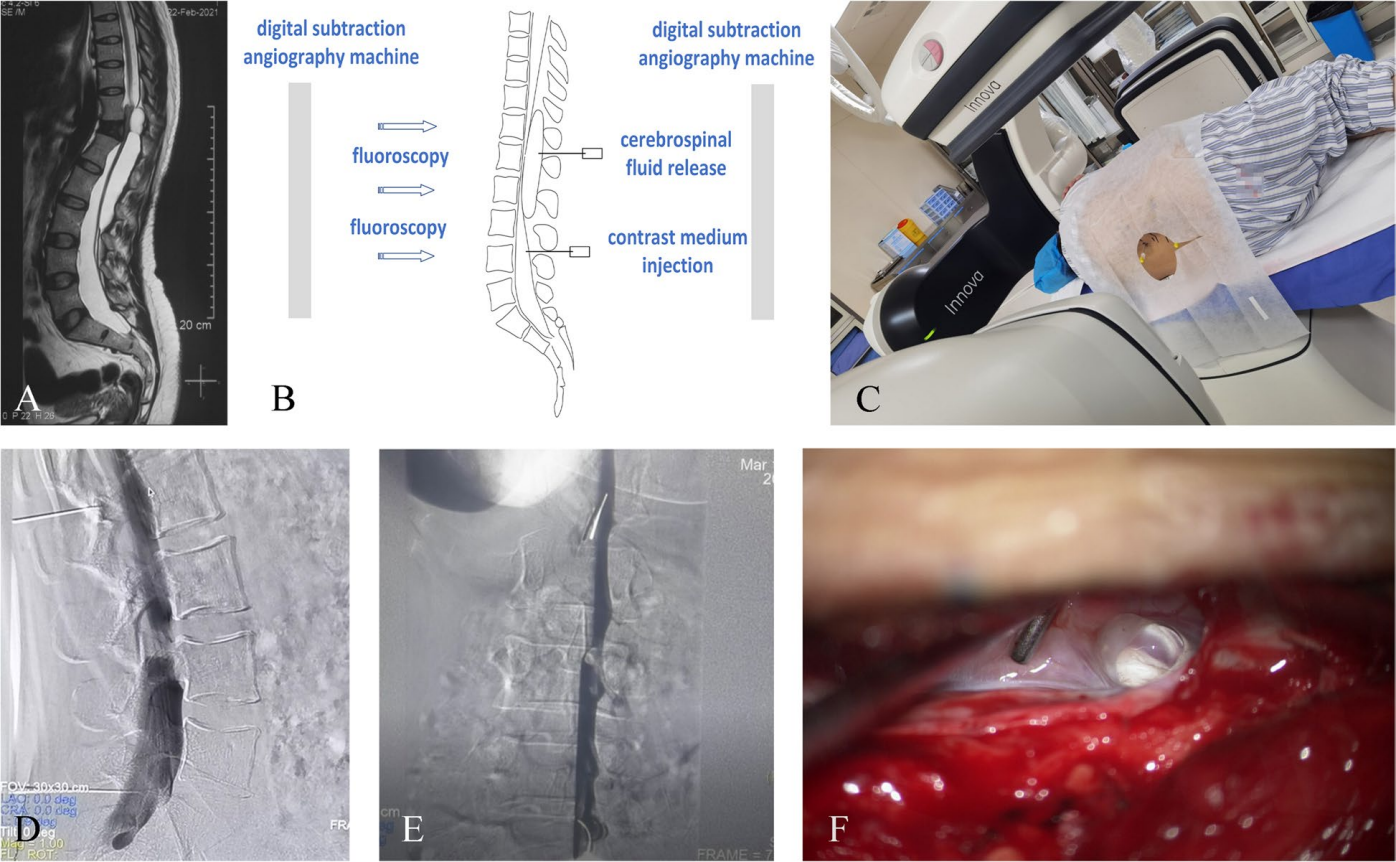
Two-needle puncture digital subtraction myelography technology. A 35-year-old woman had low back pain for 4 years. A Preoperative MRI revealed a butterfly vertebra and a SEMC. B and C We performed two-needle puncture digital subtraction myelography which failed. D and E No cleft was found during digital subtraction myelography. F The cleft was found during intraoperative exploration. The drawback of these digital subtraction contrast examination techniques is that the boundary of the subarachnoid space and cyst cannot be shown
